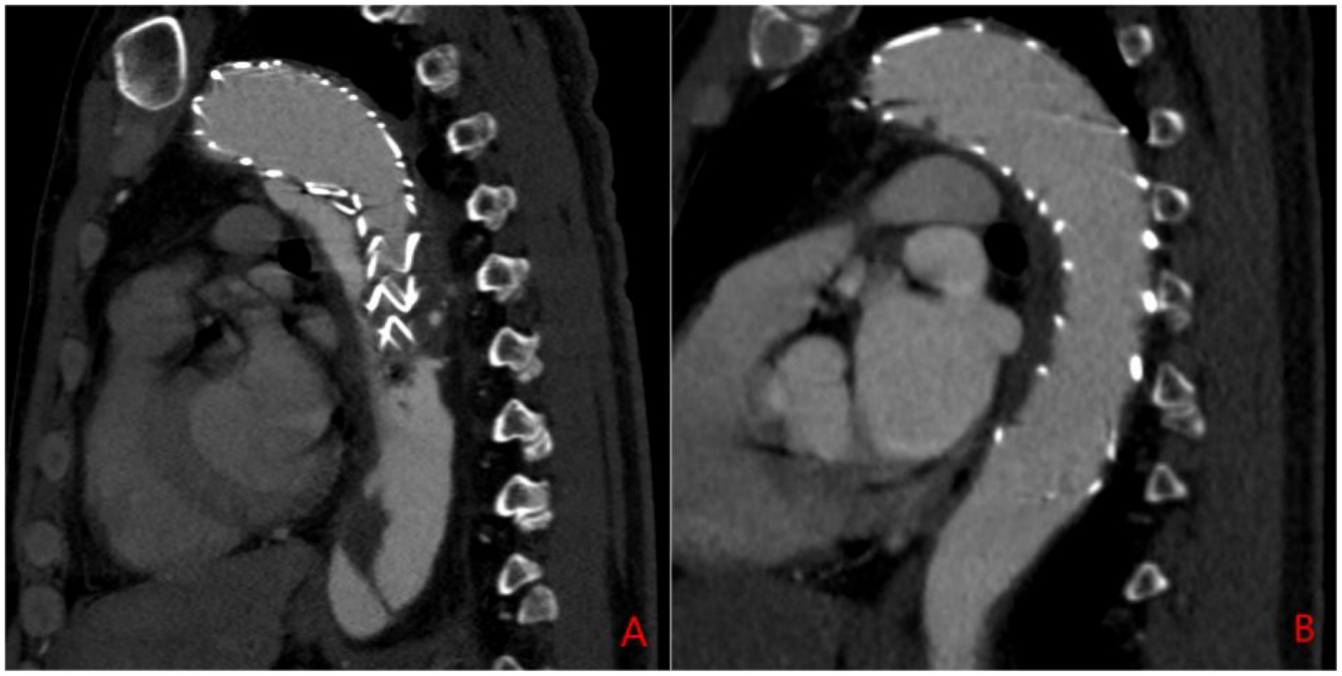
Sagittal CTA of the aorta post-TEVAR surgery. (A) Residual false lumen around the stent following TEVAR; (B) closure of the false lumen around the stent after TEVAR.
